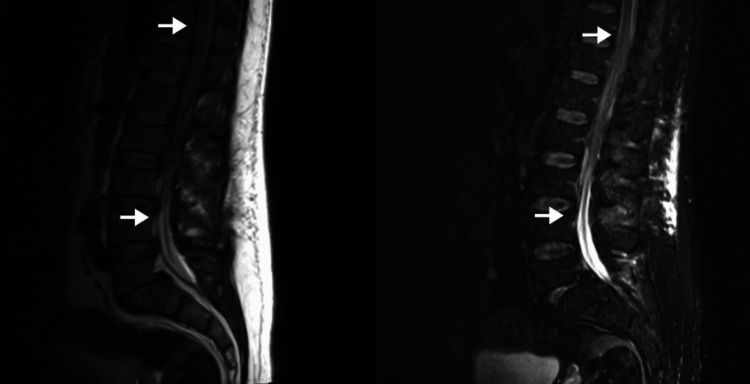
Patient’s dorsolumbar MRI showing a significant hematic collection at the subdural and anterior epidural level from D11 to L3. The arrows are delimiting the extent of the hematic collection. The top arrows show the superior part of the hematoma at the D11 level and the bottom arrows show the inferior part of the hematoma at the L3 level.
